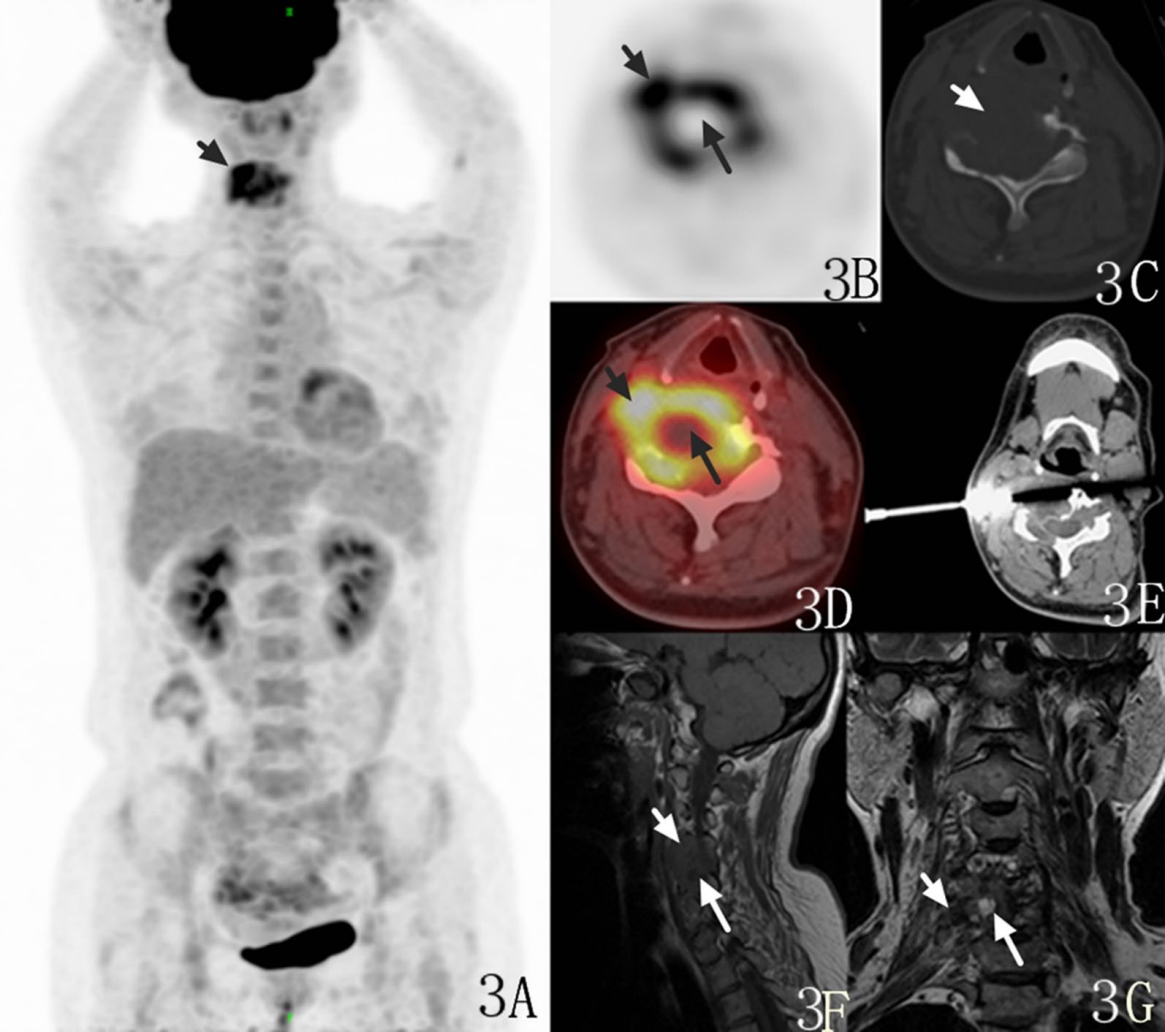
A 47-year-old female with malignant GCTTS. PET/CT maximum intensity projection (MIP) images revealed increased but heterogeneous uptake of FDG in the C4-6 vertebra (A, arrow), and no other lesions were found in any part of the body. The spinal lesion shows heterogeneous FDG uptake with central deficiency (B, D, long arrow) but higher uptake in the periphery (B, D, short arrow), with an SUVmax value of 8.9. CT showed irregular osteolytic bone destruction (C, arrow). CT-guided biopsy was performed on the hypermetabolic area of the lesion (E). The lesion showed signal hypointensity on sagittal T1WI image with unclear margin (F, short and long arrows) and hyperintensity in the central region but hypointensity in the periphery on coronal T2WI images (G, short and long arrows)
